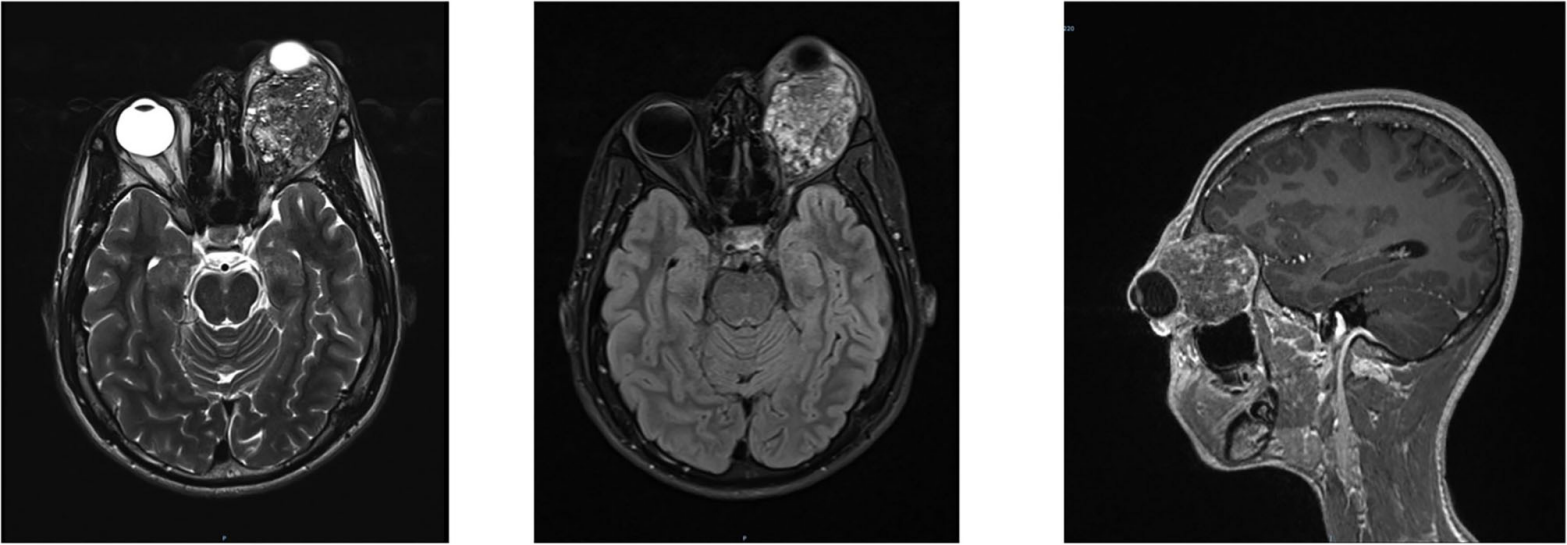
Magnetic resonance imaging of brain and orbits, with T1- and T2-weighted sequences, showed the presence of a bulky pathological tissue located in the left retrobulbar intraorbital space, displacing anteriorly the eyeball and incorporating the optic nerve, which did not appear recognizable in all its intraorbital segments.
